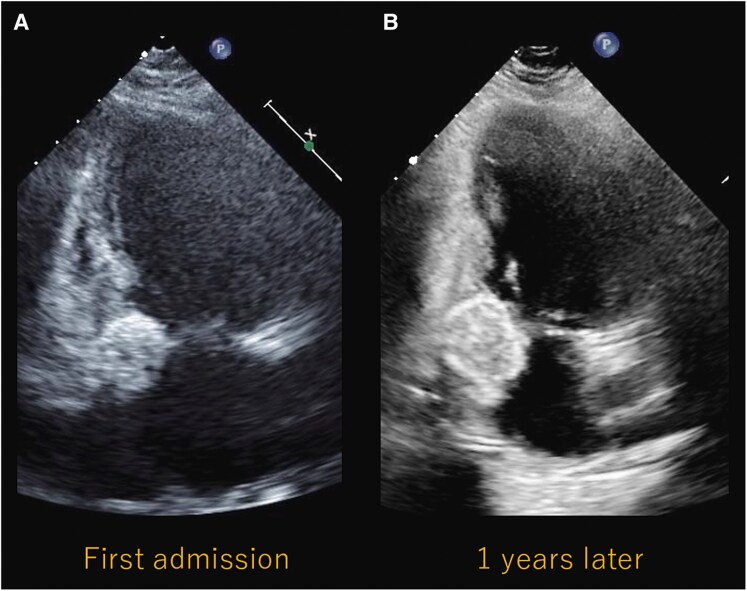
Transthoracic echocardiogram at presentation (*A*) and 1 year later (*B*). These are from the apical two-chamber view. The mass had increased in size from 17 × 19 mm to 23 × 26 mm.
